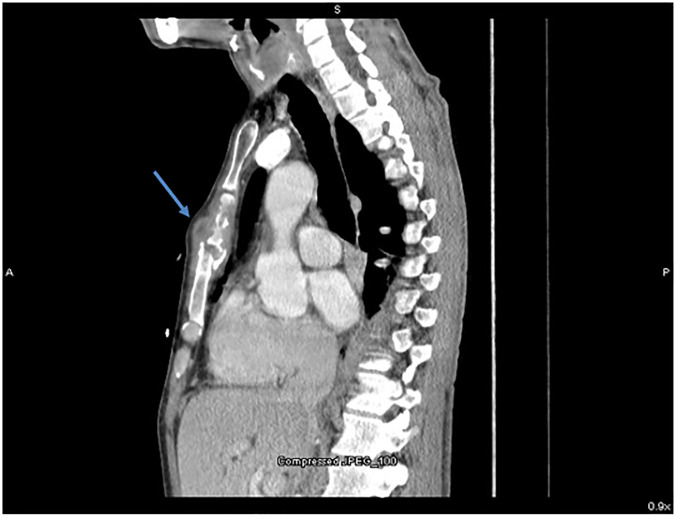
Computed tomography of the chest with contrast (sagittal view) showing fluid enhancing mass overlying the sternum with bony destruction of the sternum (blue arrow).
